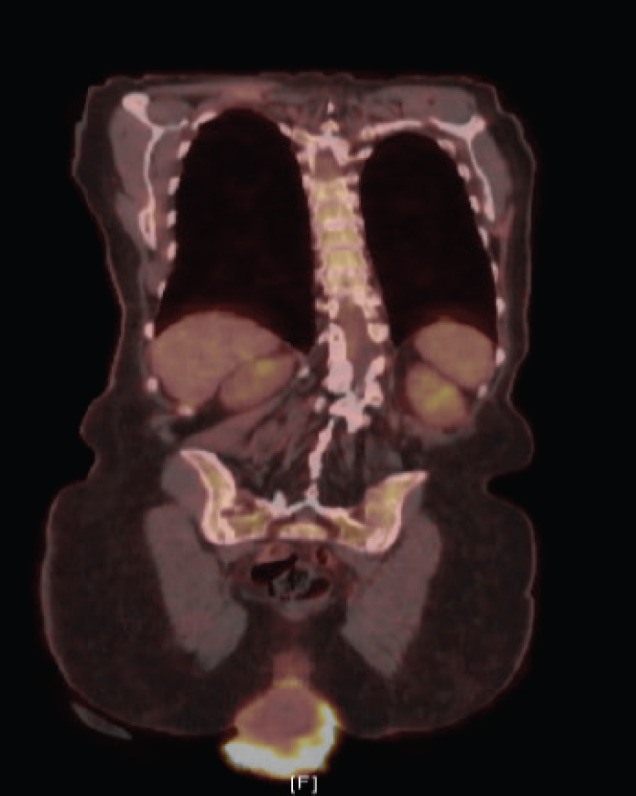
PET/CT demonstrating severe anterior, middle, and posterior compartment prolapse, with protruded mass with peripheral FDG uptake.
